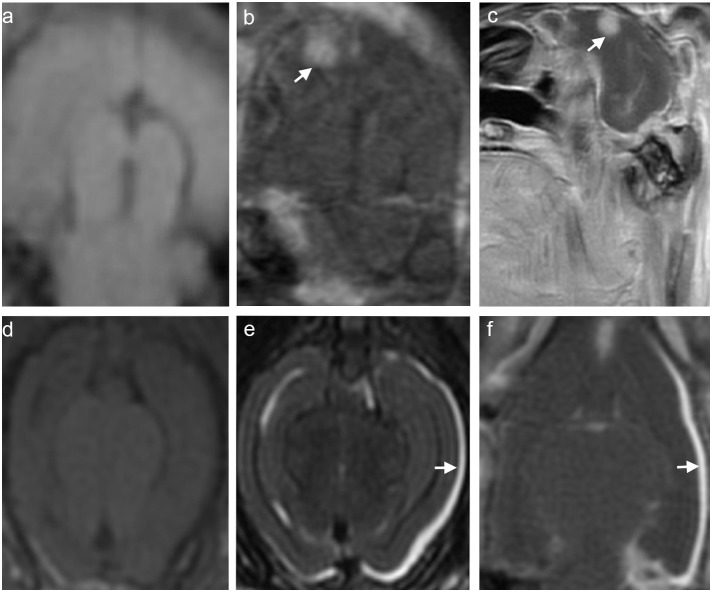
Representative magnetic resonance (MR) images of a brain parenchymal metastatic tumor (a–c) and meningeal metastases (d–f). (a) Non-contrast coronal T1-weighted (T1W) image. (b) Contrast enhanced coronal T1W image. (c) Contrast enhanced sagittal T1W image. (d) Non-contrast axial T1W image. (e) Non-contrast axial T2-weighted image. (f) Contrast enhanced coronal T1W image. The white arrow in (b, c) and (e, f) indicate the tumor sites.
